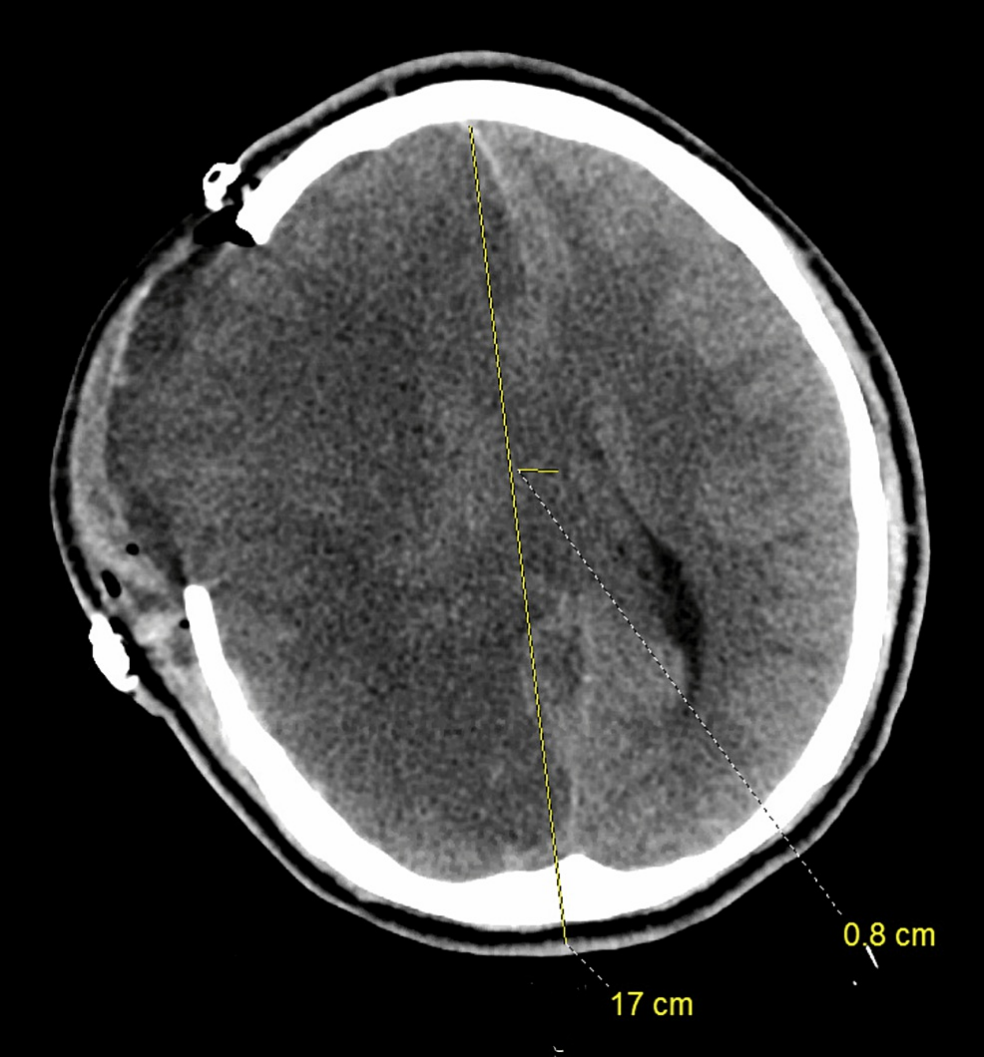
Head CT scan post decompressive craniectomy on day two shows severe cerebral edema in the right hemisphere, mass effect, and approximately 8mm right to left midline shift. CT: computed tomography
